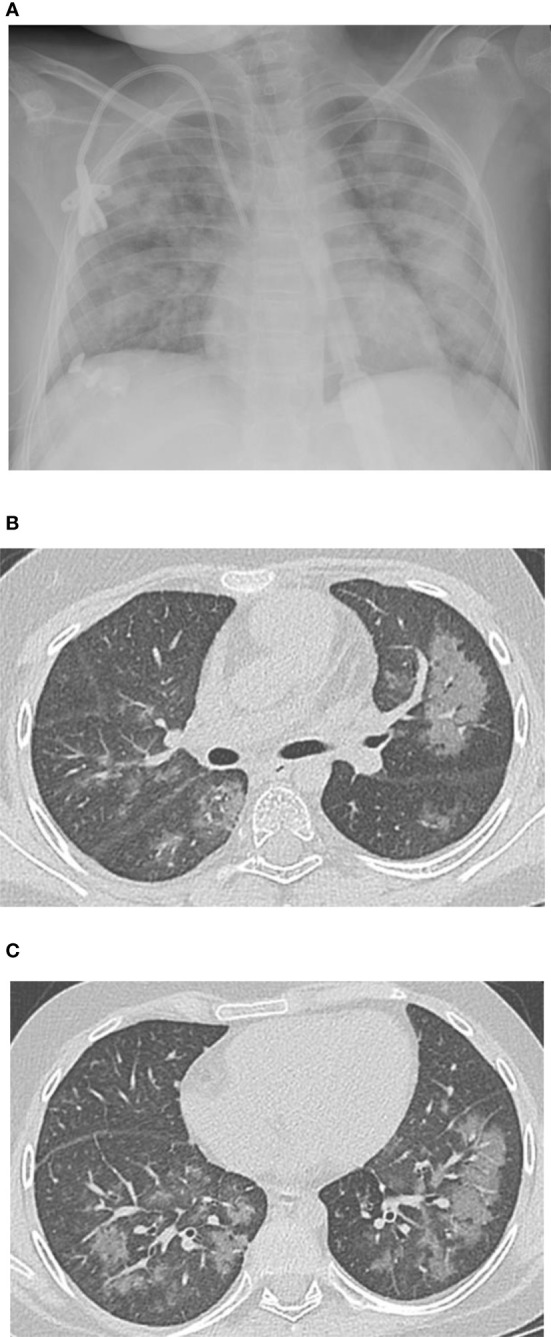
(A) Chest x-ray obtained Day +42 demonstrates diffuse airspace disease bilaterally with more focal areas of consolidation in the left lung. (B, C) Thin-section computed tomography (CT) obtained Day +49 (from 2^nd^ transplant) demonstrate diffuse ground-glass opacification involving all 5 lobes.
